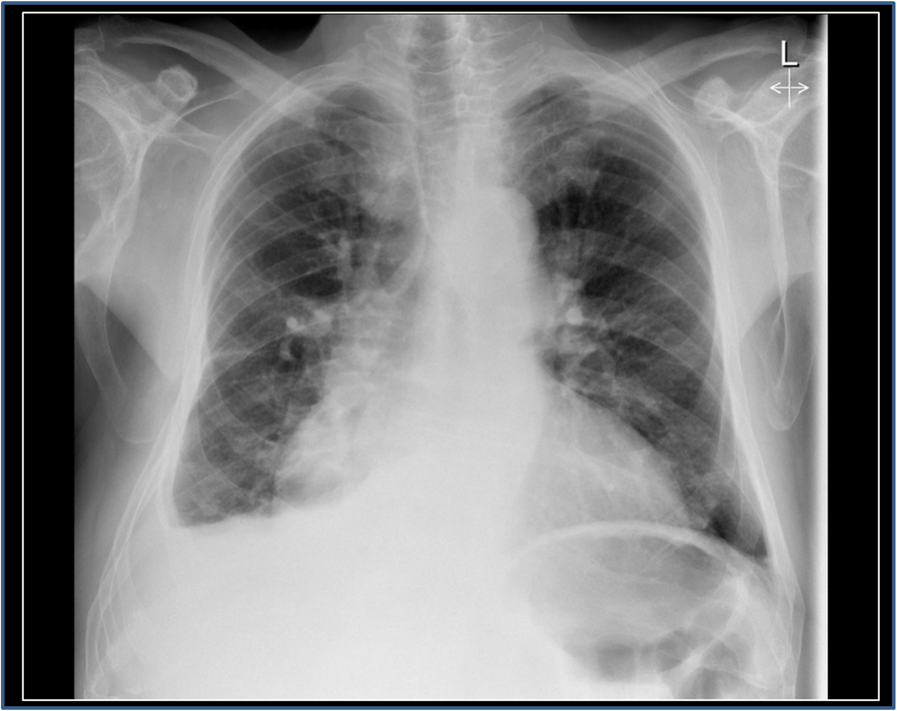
Chest X-ray depicting a global enlarged heart, chronic pulmonary congestion, and right-sided pleural effusion
